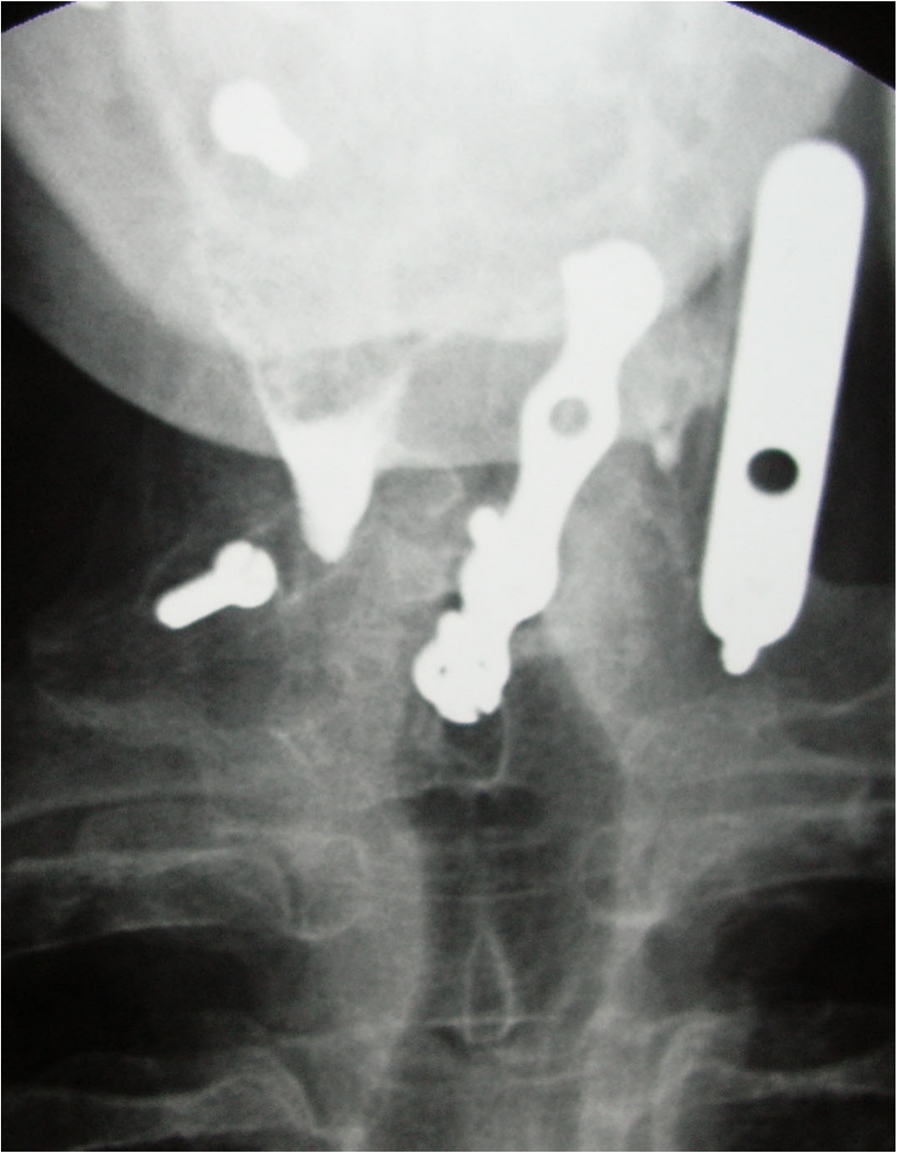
Radiograph showing the absence of the mobilized screw in the anterior plate
